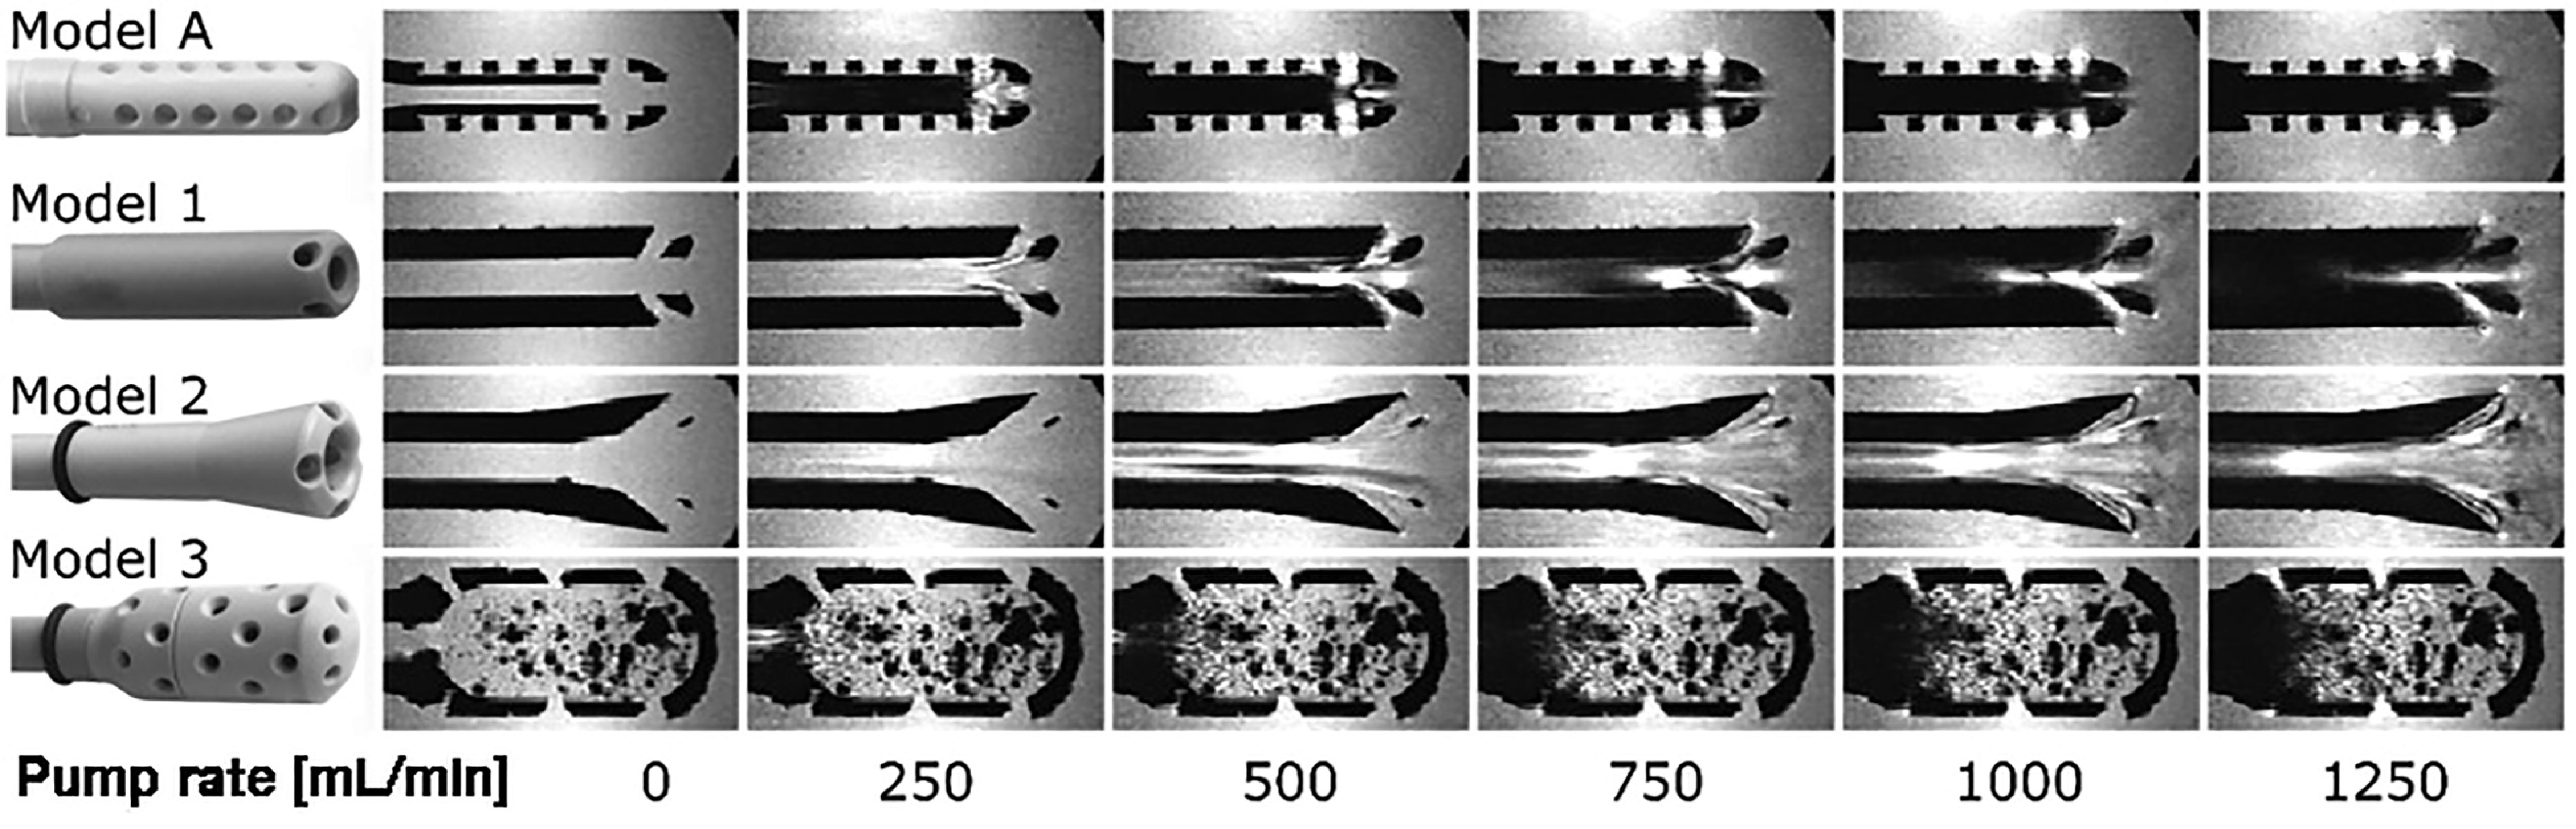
T1-weighted images of surgical suction heads in water. Increasing flow rates introduce signal void indicating areas of turbulent flow.
